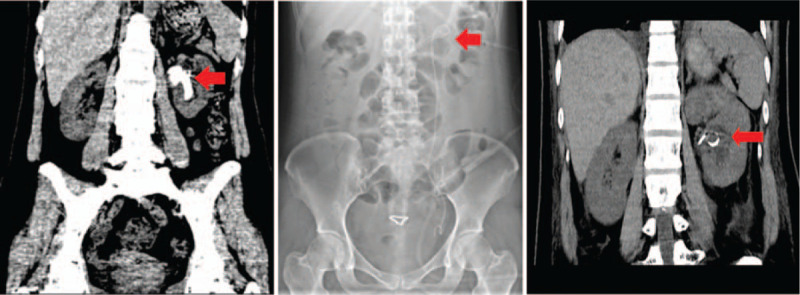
Imaging data of the patient's first hospitalization in the authors’ department. Left panel, the computed tomography (CT) scan showed multiple stones in the left kidney and hydronephrosis after admission on April 13, 2020. Postoperative reexamination of the plain abdominal radiograph (Middle panel) and CT scan (Right Panel) revealed that the left kidney stones were basically cleared on April 22, 2020.
